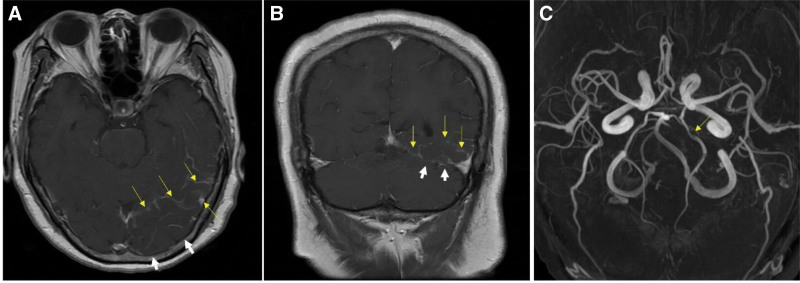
Brain MRI and MRA at the patient’s second visit. Brain MRI reveals gyral swelling in the left temporo-parieto-occipital lobes (white arrow) and sulcal vascular enhancement (yellow arrow) around the swelling in the T1 axial view (A) and T1 coronal view (B). Brain MRA reveals focal stenosis on the left proximal posterior cerebral artery (yellow arrow), which does not seem significant enough to cause homonymous hemianopia. MRA = magnetic resonance angiography, MRI = magnetic resonance imaging.
